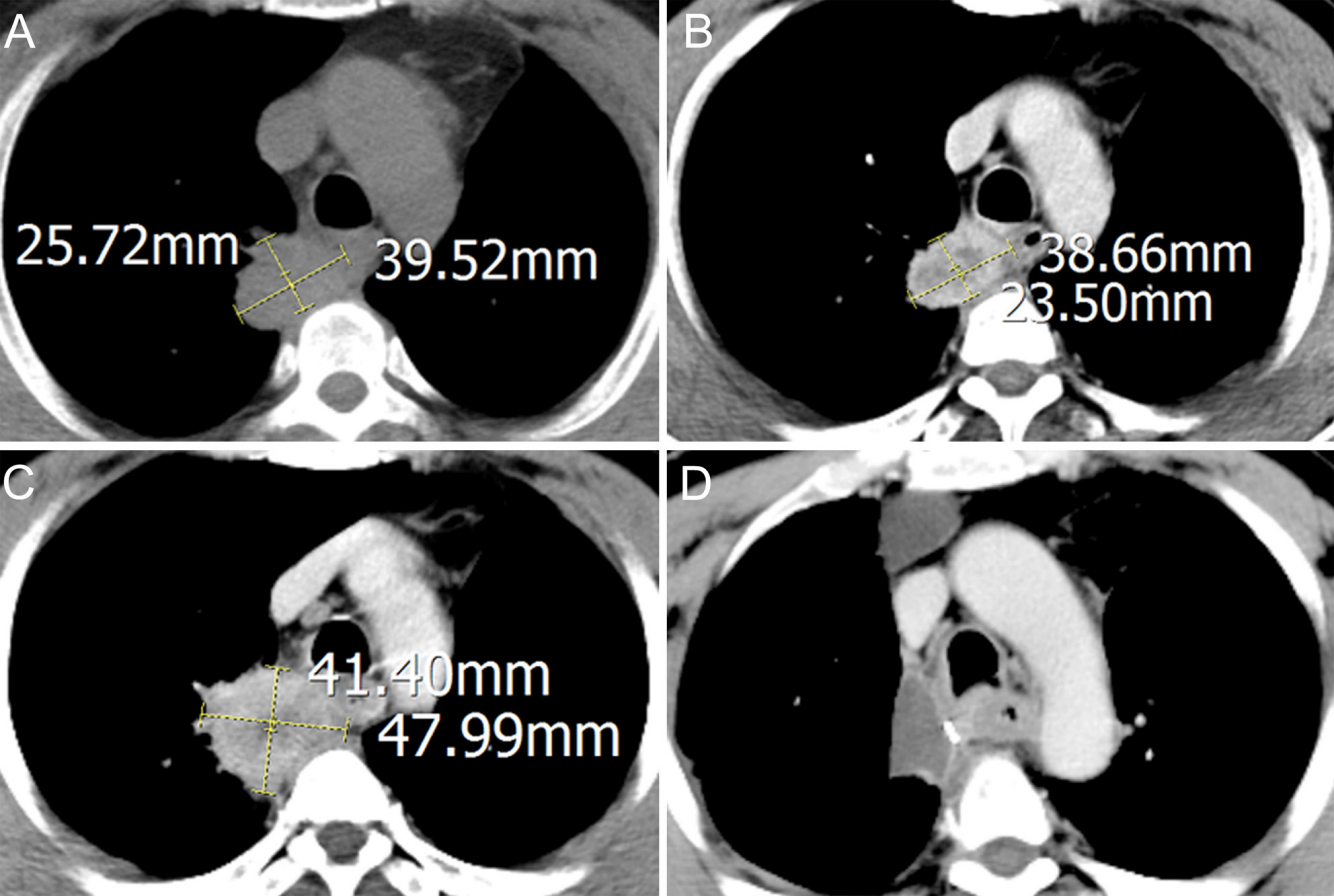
Computed tomography (CT) imaging of primary lesion at different times: (A) treatment-naïve; (B) after two cycles of neoadjuvant chemotherapy; (C) after another two cycles of neoadjuvant sintilimab combined with chemotherapy; (D) after operation.
